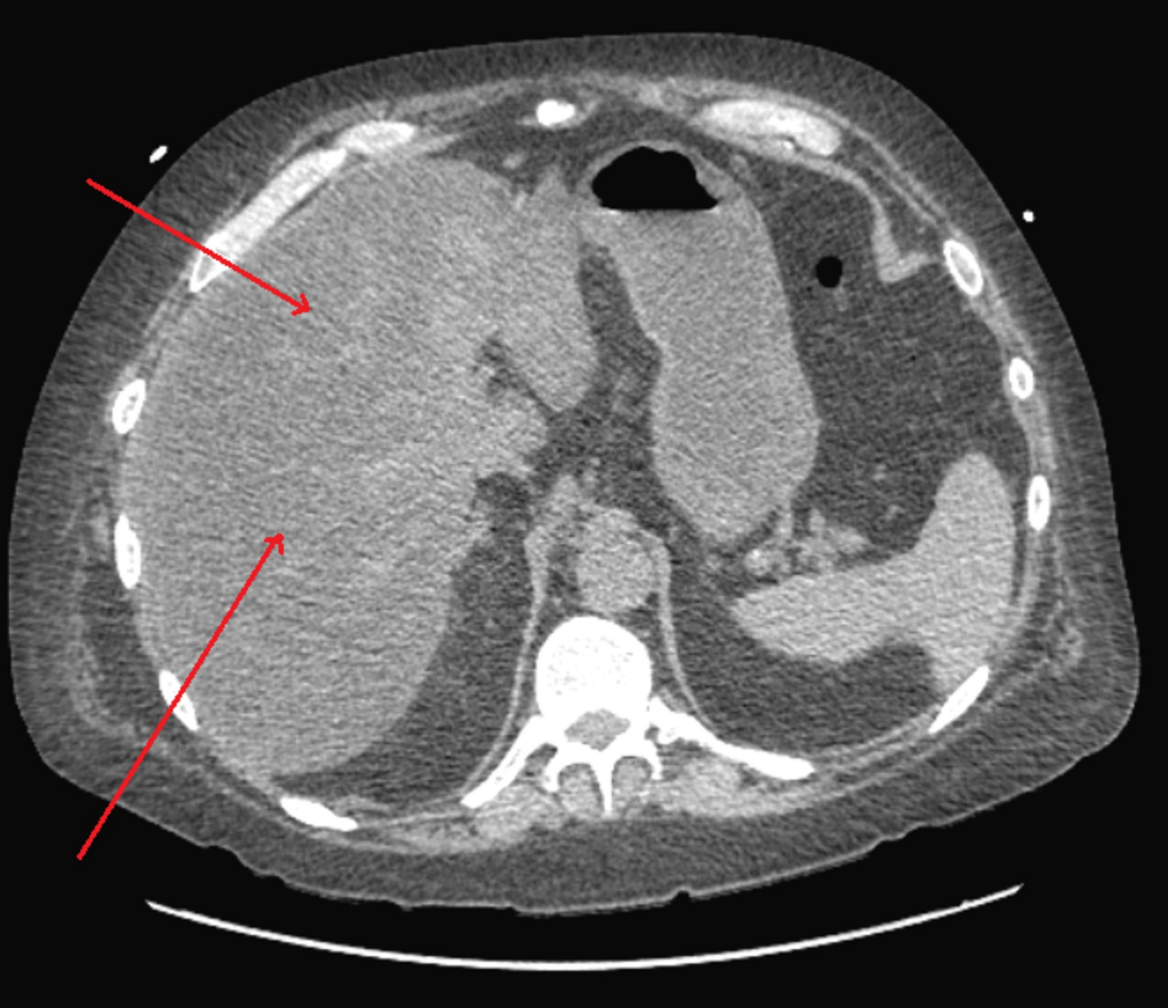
CT of the abdomen showing diffuse hepatic steatosis
